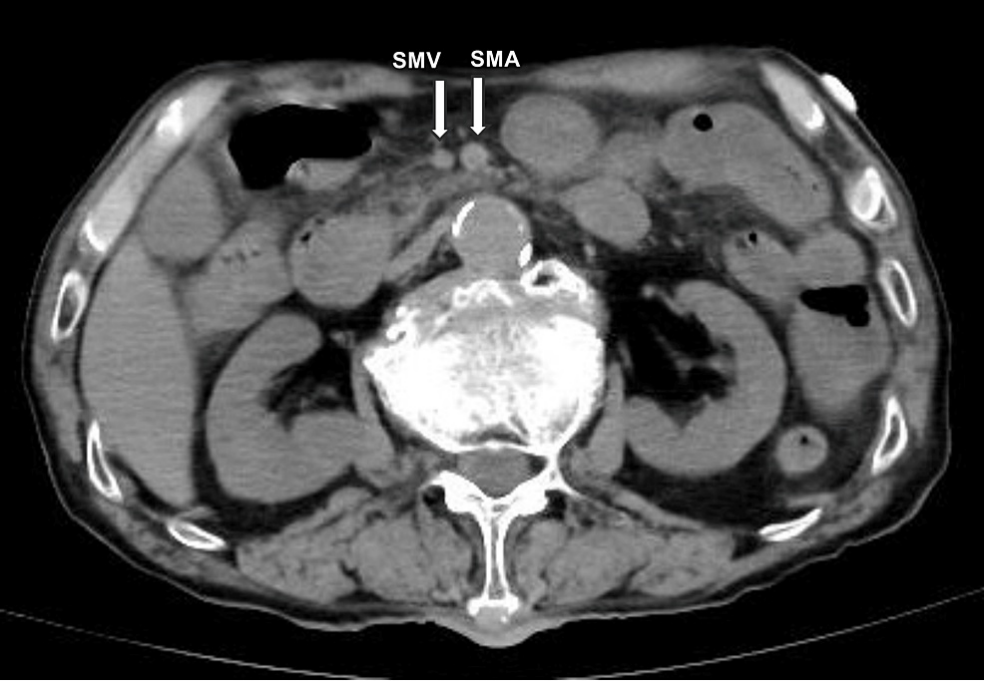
Smaller SMV sign shown on abdominal CT SMA: superior mesenteric artery, SMV: superior mesenteric vein
